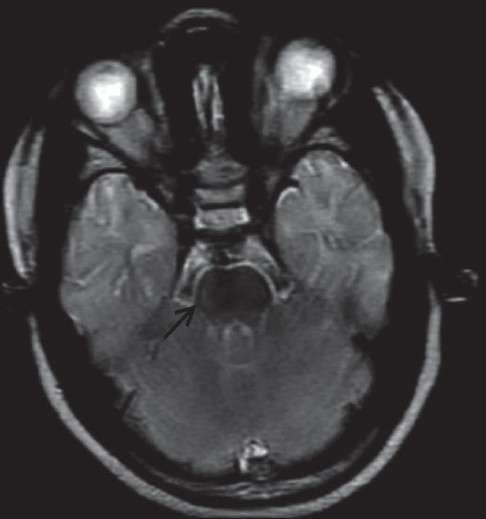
T2W axial magnetic resonance image in a 10-year-old child showing subtle hyperintense lesions in the pontine white matter (arrow)
